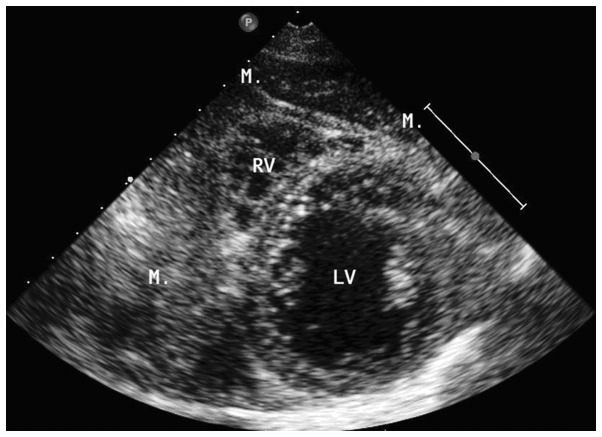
Parasternal short axis ultrasound showing a large mass abutting the left and right ventricle on the right, anterior and left epicardium surfaces. M, mass; RV, right ventricle; LV, left ventricle.
